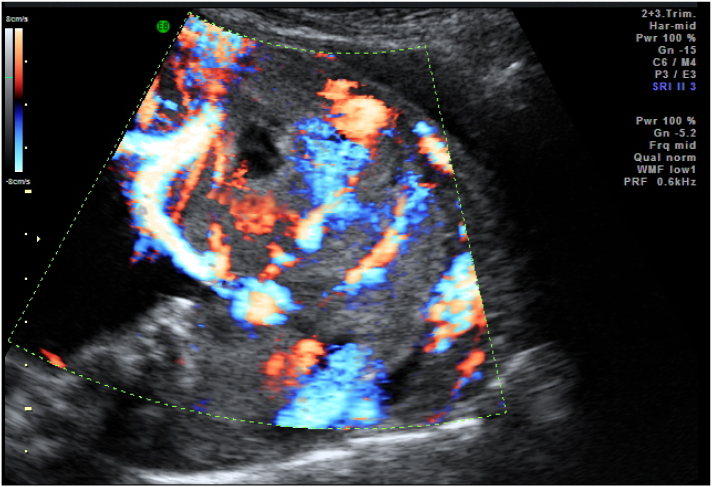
Placenta accreta spectrum ultrasound.
